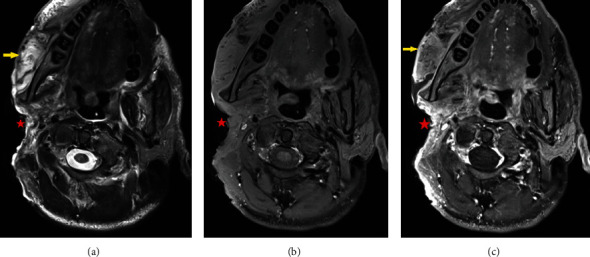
Axial MRI of the head showing postoperative outcome by after the first stage of debulking with a drastic reduction in tumour tissue (red star). (a) T2-weighted imaging, (b) T1-weighted imaging, and (c) T1-weighted imaging after gadolinium injection. The masticator muscles show increased T2w intensity and diffuse enhancement, due to muscle oedema (yellow arrow). Residual tumour tissue is difficult to differentiate for which follow-up imaging is necessary.
